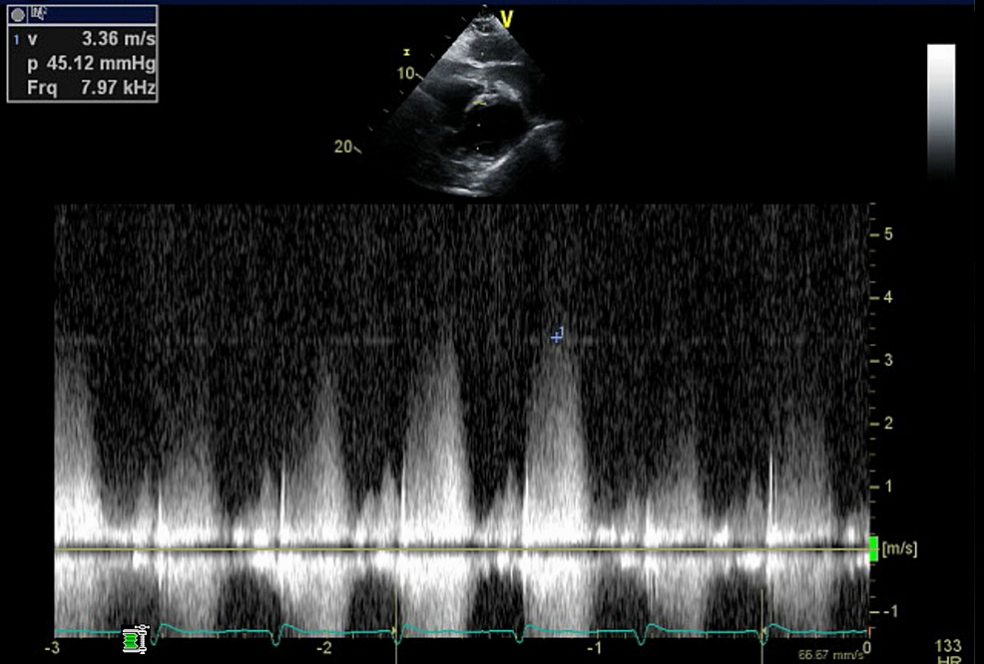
Doppler waveforms measure velocity and pressure gradient across ventricular septal defect
